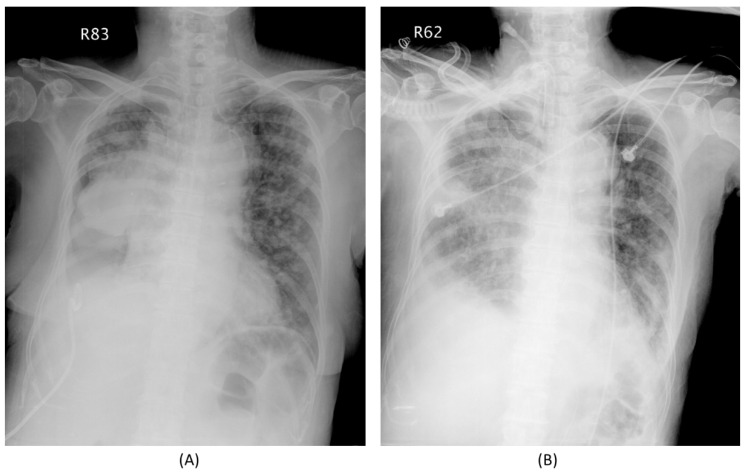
Case of a patient who experienced a dramatic response to EGFR-TKI during ICU treatment. This 72-year-old woman had right lung adenocarcinoma, cT4N3M1c, stage IVB, with malignant pleural effusion, lung-to-lung, liver, and adrenal metastasis. She suffered from an episode of pneumonia and hypercapnic respiratory failure, and was admitted to the ICU for intensive care. The plain film before (A) and 2 weeks after gefitinib (B) are shown. The patient finally was weaned successfully from the mechanical ventilator and discharged home with tracheostomy under ambient air. Acronyms: EGFR = epidermal growth factor receptor, ICU = intensive care unit, TKI = tyrosine kinase inhibitor.
